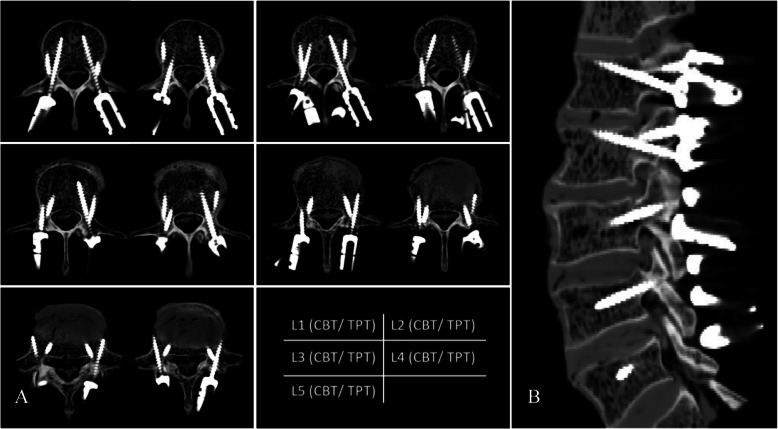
Lumbar CT scan showing that the positions of the TPT and CBT screws were satisfactory on the transverse plane a and the sagittal plane b of the L1-L5 vertebral body segments
